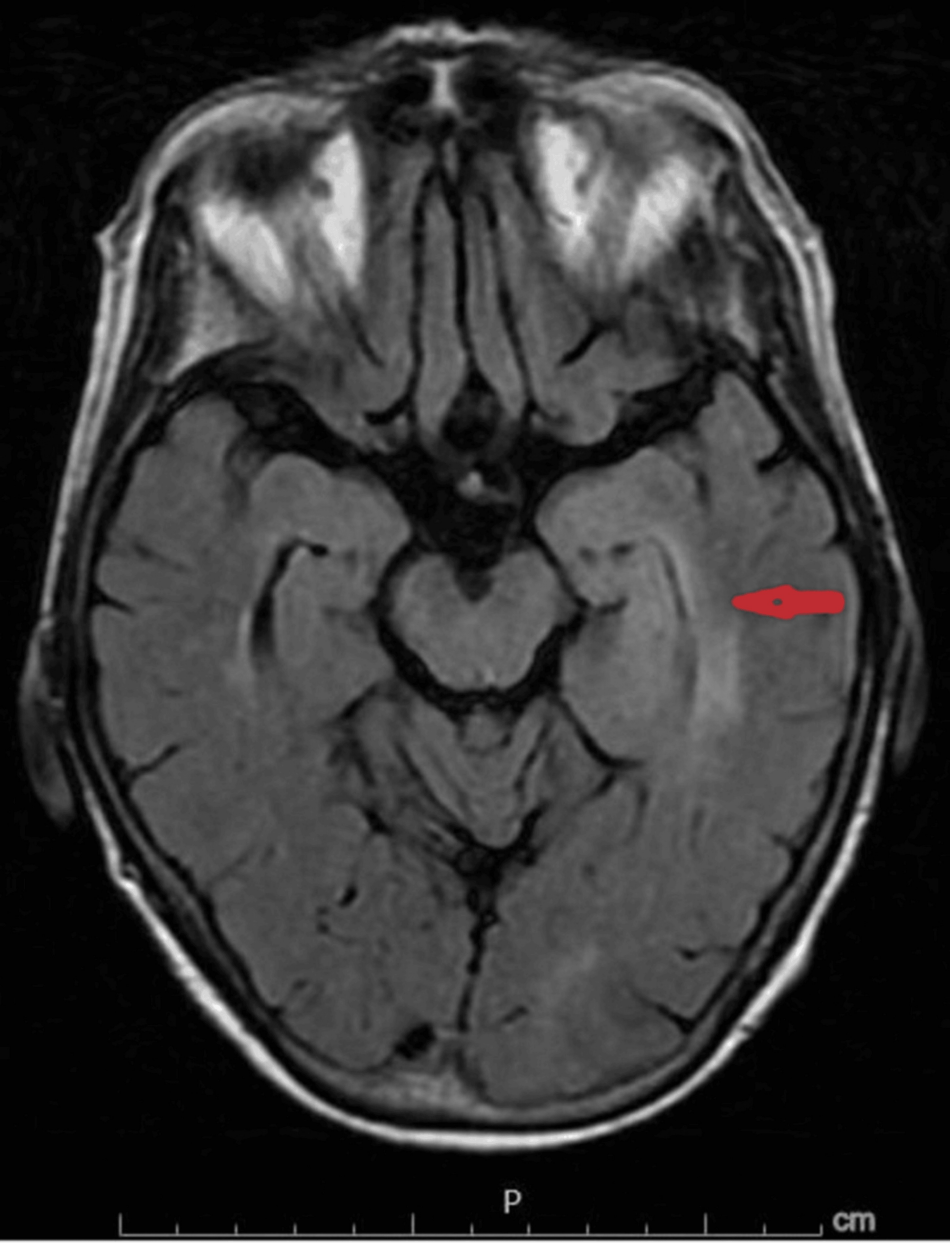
MRI of the brain with and without IV contrast shows mild asymmetric fullness and FLAIR hyperintensity about the medial left temporal lobe/hippocampus without postcontrast enhancement or midline shift. Findings are nonspecific and could relate to infectious etiologies such as herpes simplex encephalitis versus unilateral autoimmune encephalitis. MRI, magnetic resonance imaging; FLAIR, fluid-attenuated inversion recovery
